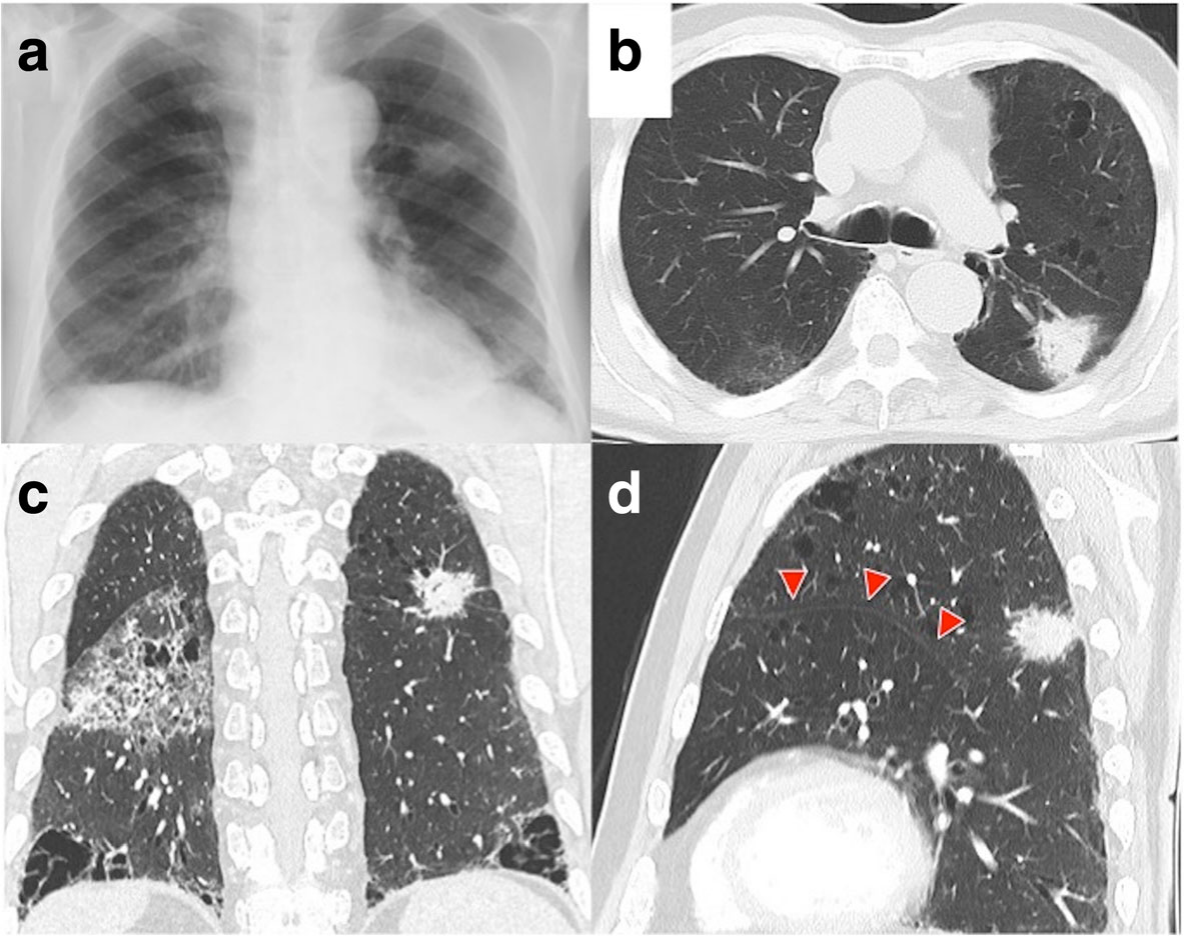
a, b Chest radiograph and CT image showing a mass in the left lung S^1 + 2^ segment. c Chest CT image showing a mass, emphysema in the upper lobes, and fibrosis in the lower lobes. d Chest CT image showing an accessory fissure between S^1–3^ and S^4 + 5^ (arrowheads)
